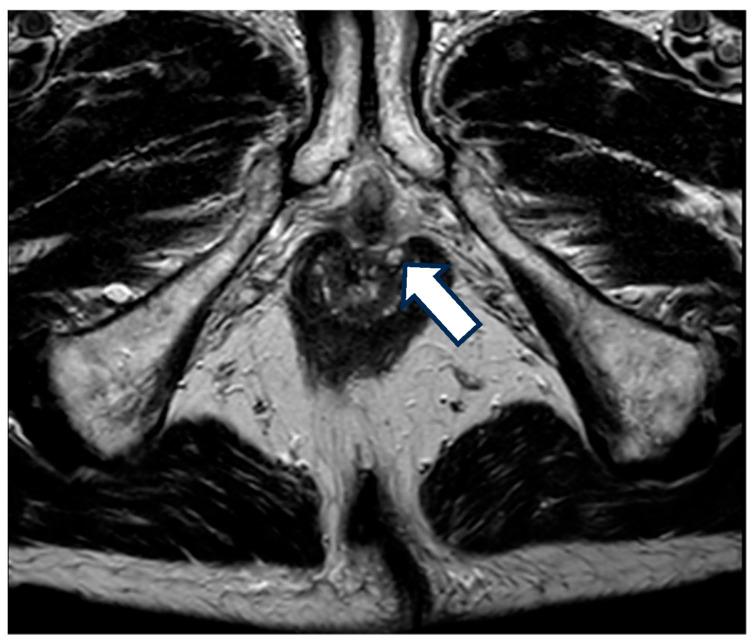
MRI with fistula. A 68-year-old patient with a 20-year history of Crohn’s disease and a prior diagnosis of perianal Crohn’s disease (PCD) presented with anal pain. Upon examination, a perianal ulceration approximately 3 cm in diameter was observed, and medical treatment with antibiotics was initiated. A pelvic MRI was requested for further characterization, which identified several fistulous tracts, without the identification of the location of the anal ulcer. The arrow in the image is pointing towards one of the fistulous tracts.
